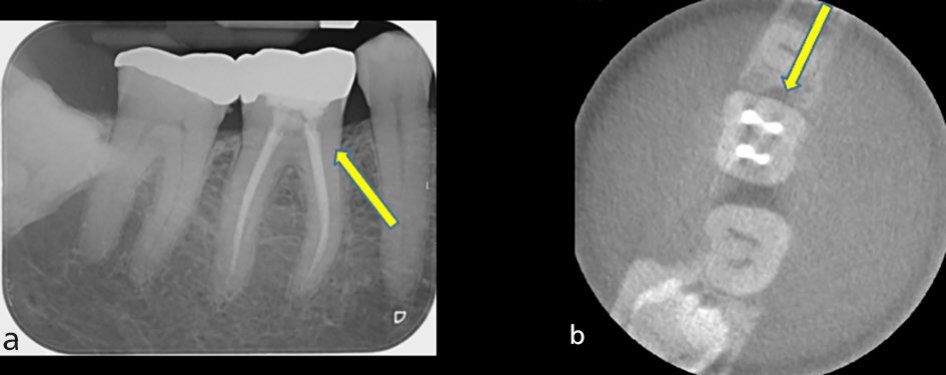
Five years after the completion of root canal treatment and crown placement, this mandibular right first molar has developed symptoms. No isolated periodontal probing depths were evident. a) Although, there is no evidence of emerging disease evident with conventional radiographic imaging. b) Small volume CBCT demonstrates a localised area of mesial crestal bone loss, consistent with a vertical root fracture. Image courtesy of Dr Noushad Rahim
